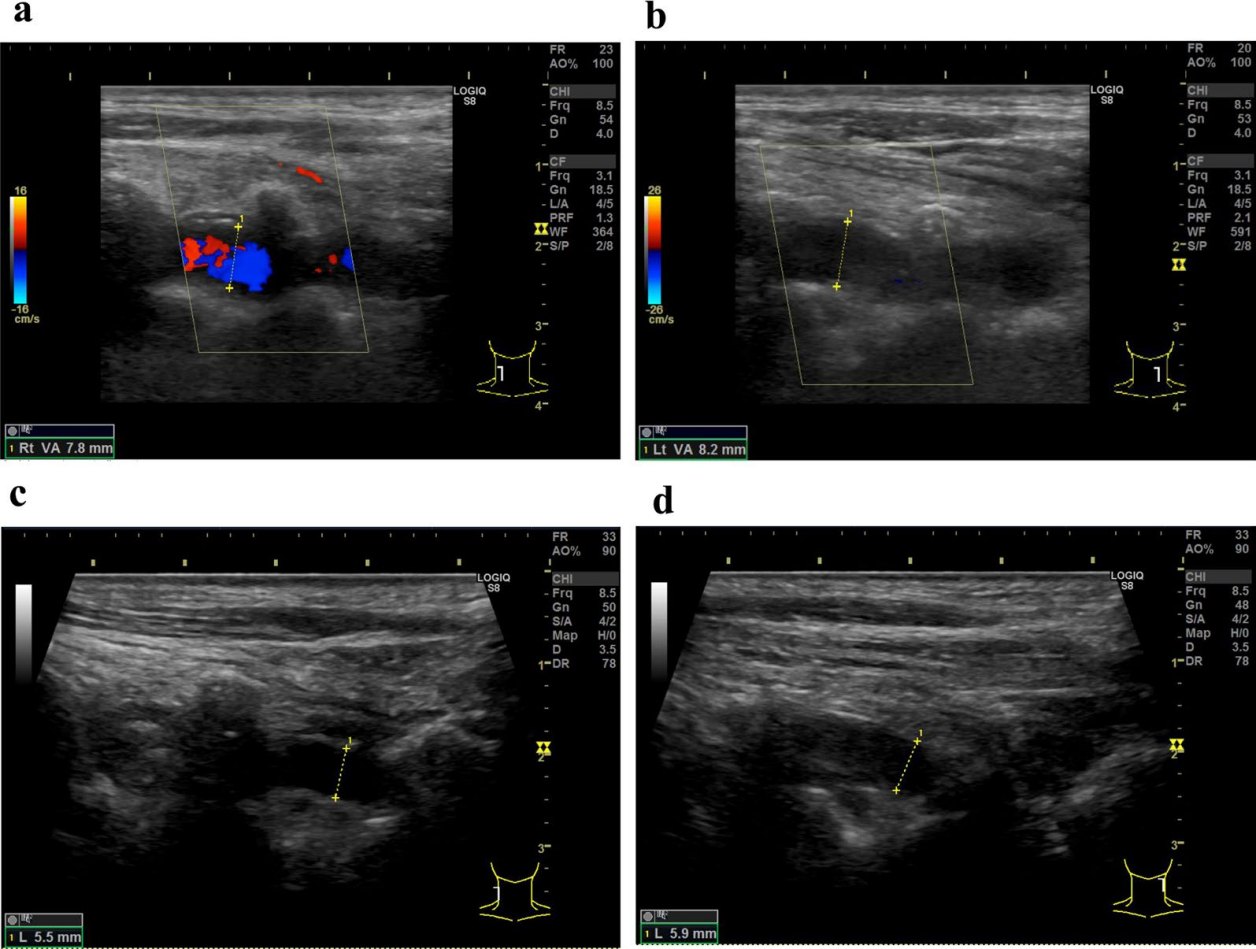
Ultrasound findings of bilateral vertebral arteries before and after treatment. Bilateral vertebral arteries were observed from bifurcation to C3 level by ultrasound. Before treatment, those were dilated to approximately 8 mm on all levels. a Right vertebral artery. b Left vertebral artery. Four years and 8 months after treatment, the above-mentioned arteries decreased to approximately 5.5 mm on all levels. c Right vertebral artery. d Left vertebral artery
